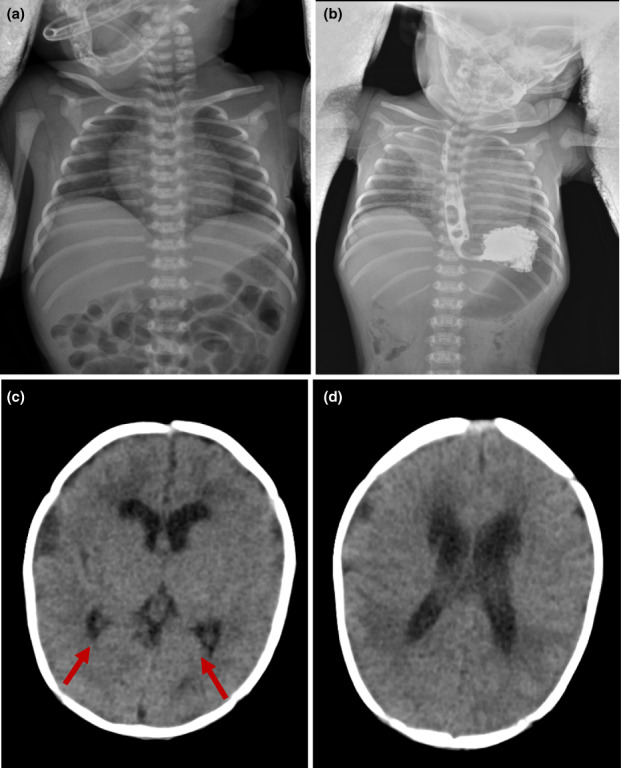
(a) Chest radiographs: Bilateral diaphragmatic lifts. (b) Gastrointestinal angiography: Unobstructed esophagus, gastroesophageal reflux. (c) Head‐CT: Bilateral subependymal cysts. (d) Head‐CT: Bilateral lateral ventricles slightly enlarged
